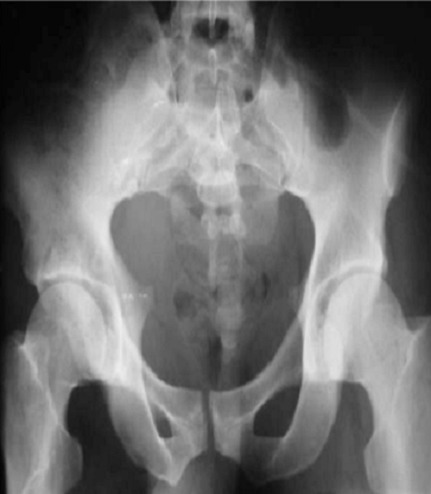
Radiographie du bassin de face après réduction de la luxation, le patient a été traité orthopédiquement
